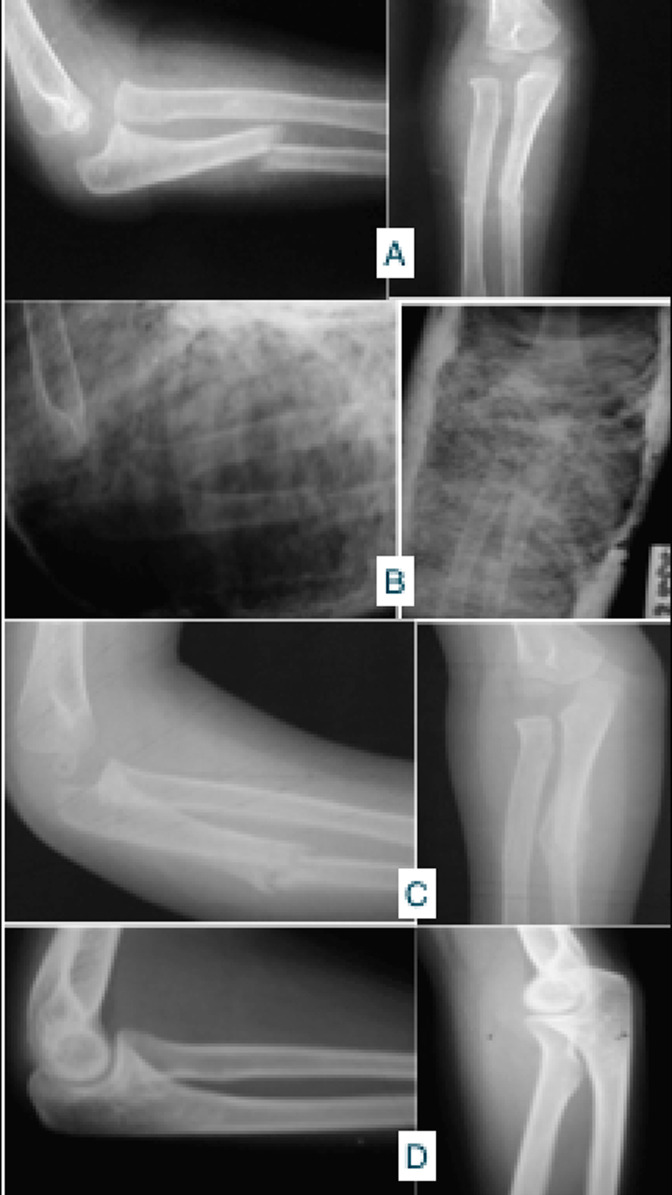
résultats après consolidation: A) radiographie face et profil de la fracture; B) radiographie face et profil après plâtre brachio anté brachial; C) résultat à un mois après un traitement orthopédique par plâtre brachio-antéro-brachial; D) résultat anatomo-radiologique à 10 ans de recul
